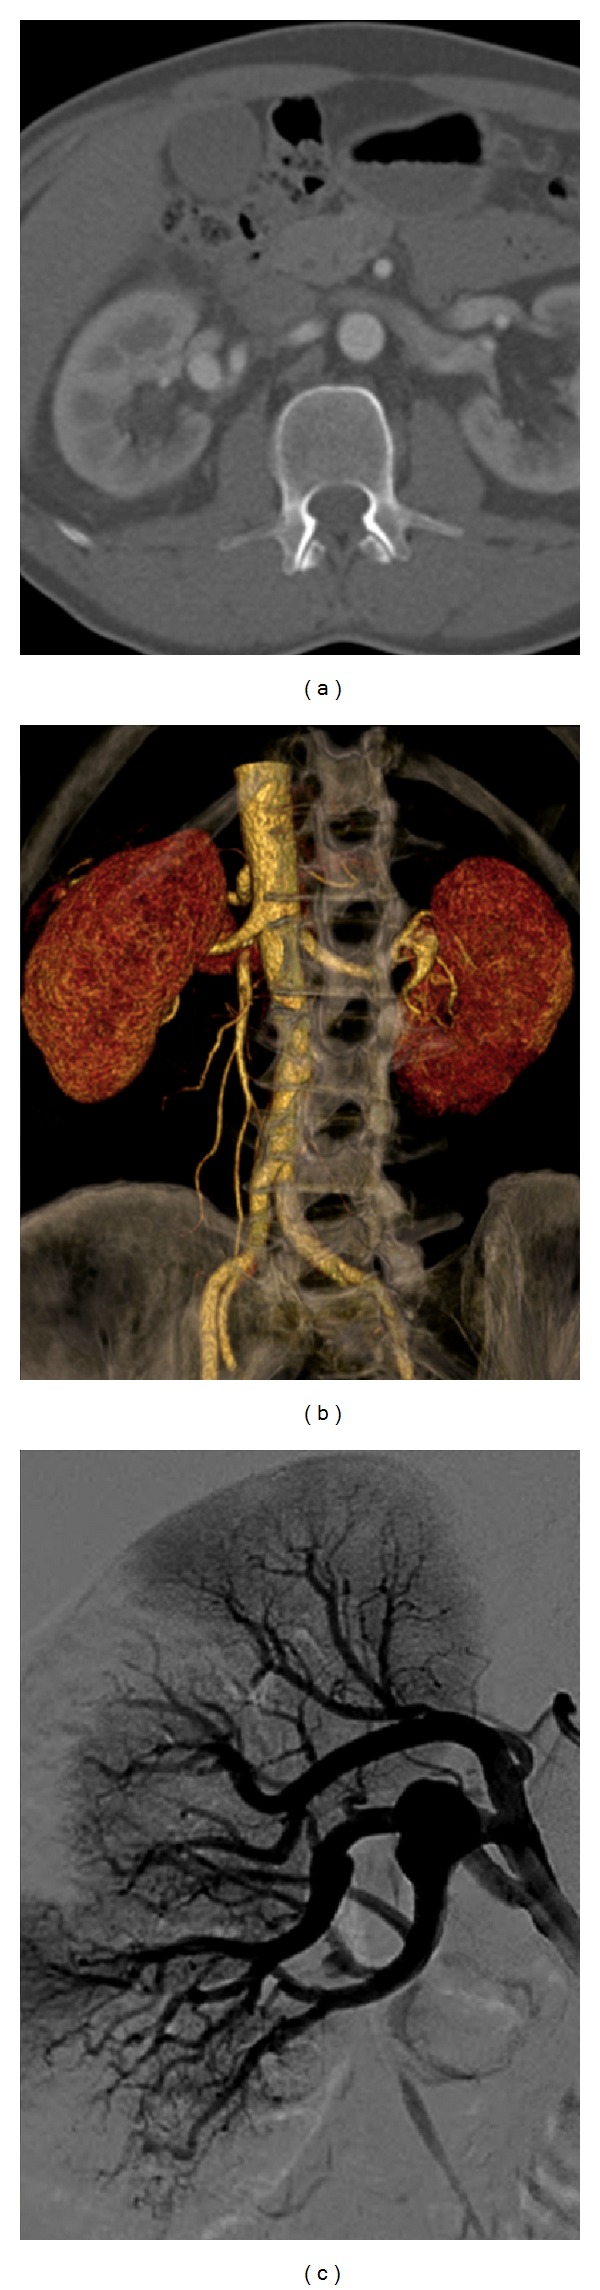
Preoperative 3D volume rendering computed tomography scan (a), with volume rendering 3D reconstruction (b) and angiography (c) showing the 20 mm fusiform aneurysm (*arrows, *a-b-c) of the secondary inferior branch of the right renal artery.
